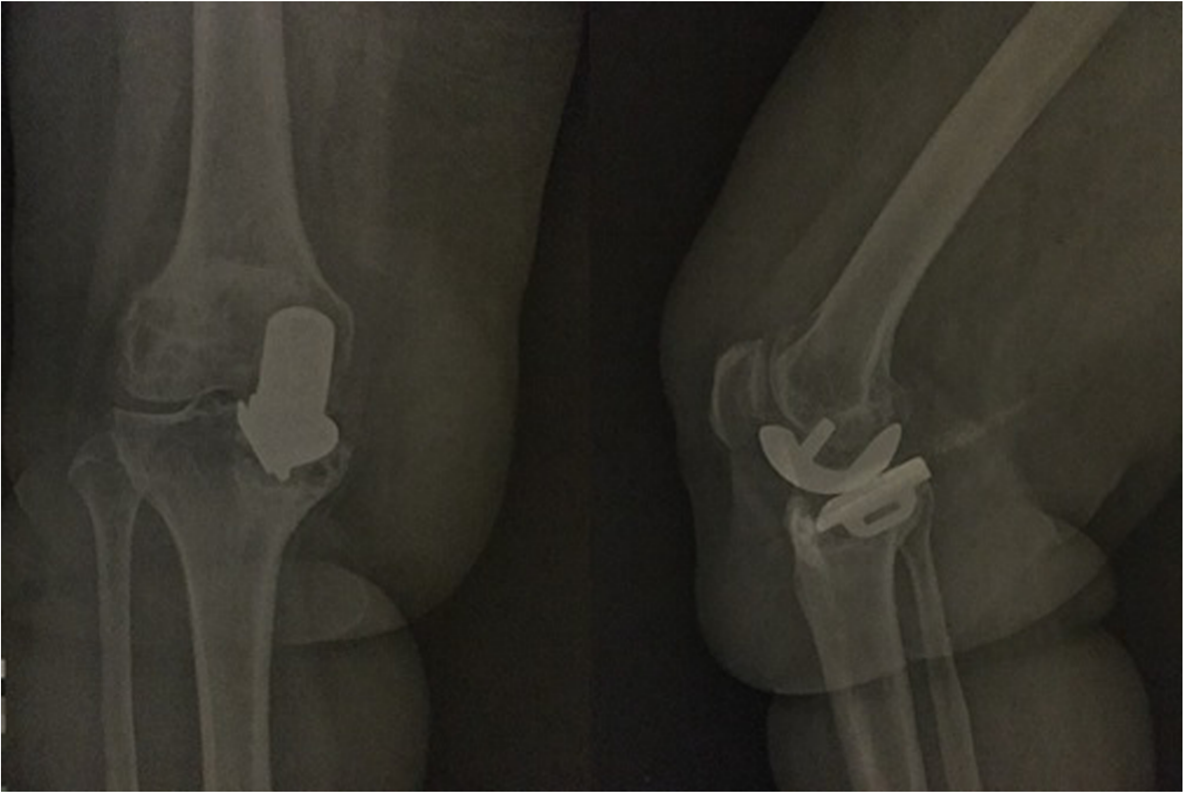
Postoperative 12 months AP and lateral X-ray of the same patient. UKA of this patient was revised at 12 months with constraint THA
